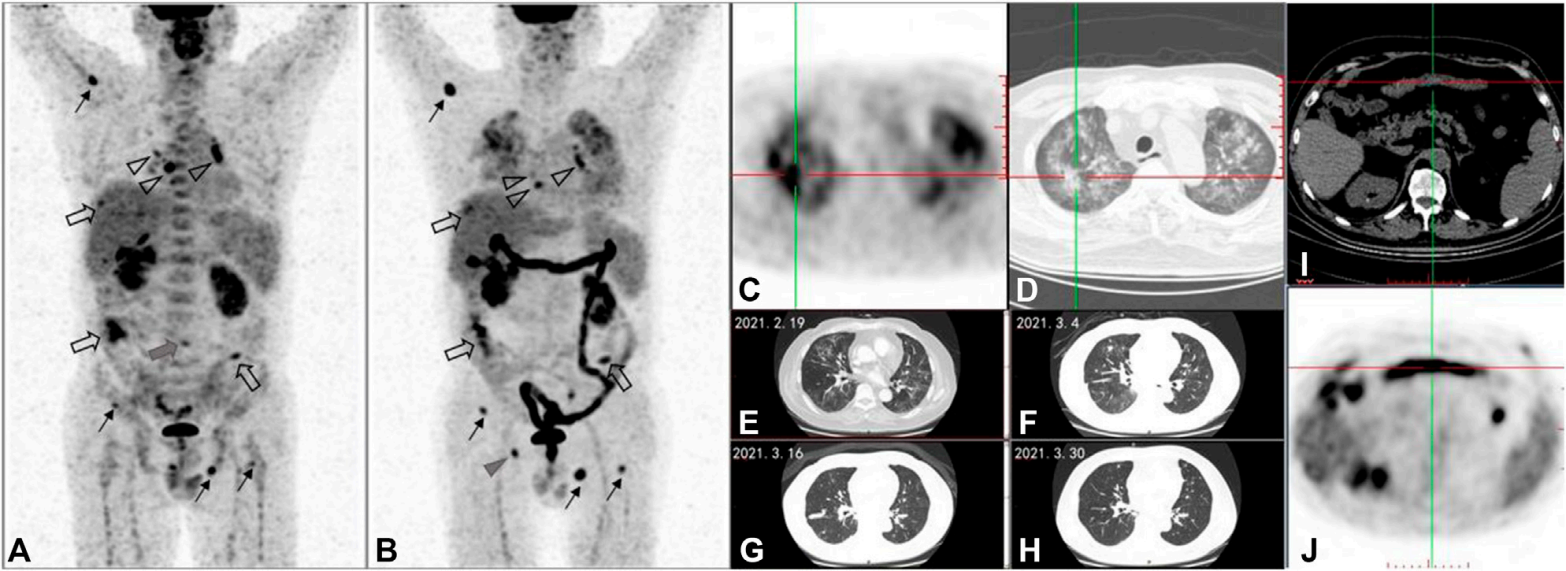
A 58-year-old male patient with metastatic intrahepatic cholangiocarcinoma was treated with the third line of ipilimumab. After four cycles of treatment, compared with ^18^F-FDG PET/CT maximum intensity projection (MIP) image (A) before treatment, the posttreatment ^18^F-FDG PET/CT (B-D), (I,J) demonstrates the following: 1) multiple soft tissue density nodules in the peritoneum, which were larger in volume and higher in FDG uptake than before ((A,B) hollow arrows); 2) multiple nodules without FDG uptake in both lungs, some of which are larger in volume than before and some of which have no change compared with before (cannot be shown in MIP images- (A,B)); 3) the lymph nodes with increased FDG uptake in mediastinal areas and bilateral hilar, which were smaller in volume and FDG-uptake lower than before ((A,B) hollow triangles); and 4) multiple foci of increased FDG uptake in bones: the FDG-uptake degree in vertebral body of lumbar 4 was significantly lower than before ((A) gray triangle); the right acetabular lesion was the new lesion ((B) gray triangle); the remaining ostial lesions were higher than those before ((A,B) black line arrows). According to PERCIMT criteron: SMD was confirmed for the number of the newly FDG-positive lesions is less than 4. Meanwhile, in the light of imPERCIST5, PMD was also evaluated for the sum of SUL_peak_ of the patient’s top five target lesions after treatment was more than 30% higher than that of the top five target lesions before treatment. As a result of clinical follow-up, the patient was confirmed PD and those lesion above were validated as metastases. PD was determined for the appearance of new lesion based on PECRIT. PMD was determined for new FDG-avid lesion at SCAN-2, which can be considered as UPMD in the line with iPERCIST. But the patient didn’t receive the next ^18^F-FDG PET/CT between days 21 and 28 after treatment or 4–8 weeks later, so it could not be evaluated according to PECRIT and iPERCIST. Follow-up results showed the PFS for ICI was 6 months. Additionally, this posttreatment PET/CT displayed: 1) newly patchy ground glass density foci in both lungs, with increased FDG uptake (C- PET axial image, D-CT axial image); 2) diffuse increased FDG uptake in ascending colon, transverse colon, descending colon, sigmoid colon and rectum (i-PET axial image) which is new compared with the previous one, and the corresponding colonic walls were not significantly thickened ((J)-CT axial image). The patchy ground glass shadows of both lungs gradually disappeared during chest CT follow-up ((E–H)-CT axial images). Combined with clinical information, the patchy ground glass shadows of both lungs were diagnosed as immunerelated pneumonia. Under the administration of MDT, the diffuse increase of glucose metabolism in the colon was considered as immune-related colitis.
